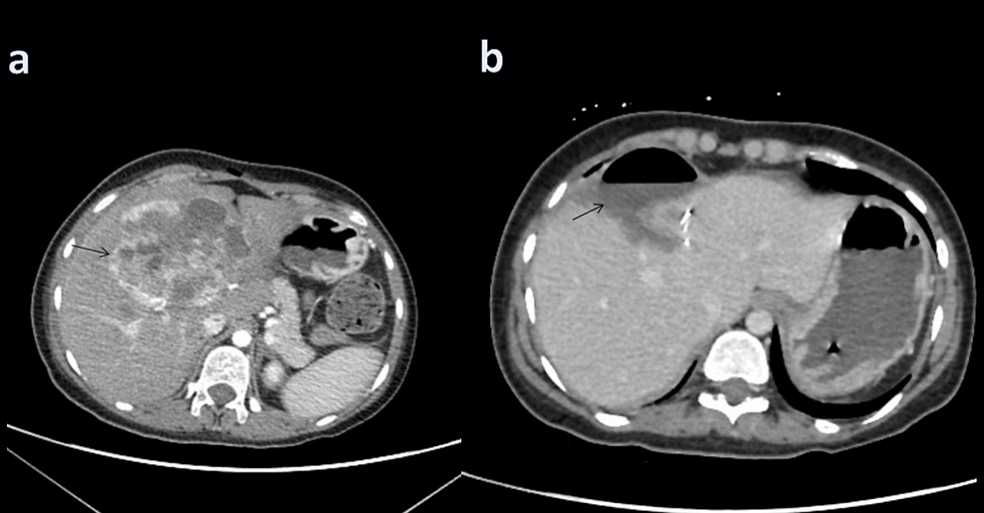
Abdominal CT scan showing (a) a large heterogeneous hypodense irregular lesion in the right liver lobe with peripheral enhancement on the arterial phase and (b) post wedge resection of the liver. Post-operative changes are noted and a fluid collection is seen anterior to the liver.
